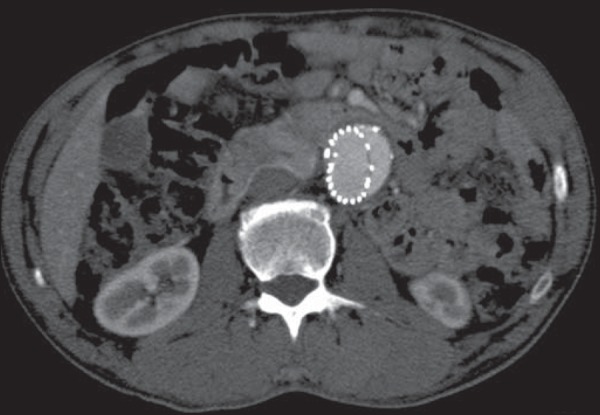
Axial section CT angiography image showing a stent in the infrarenal
